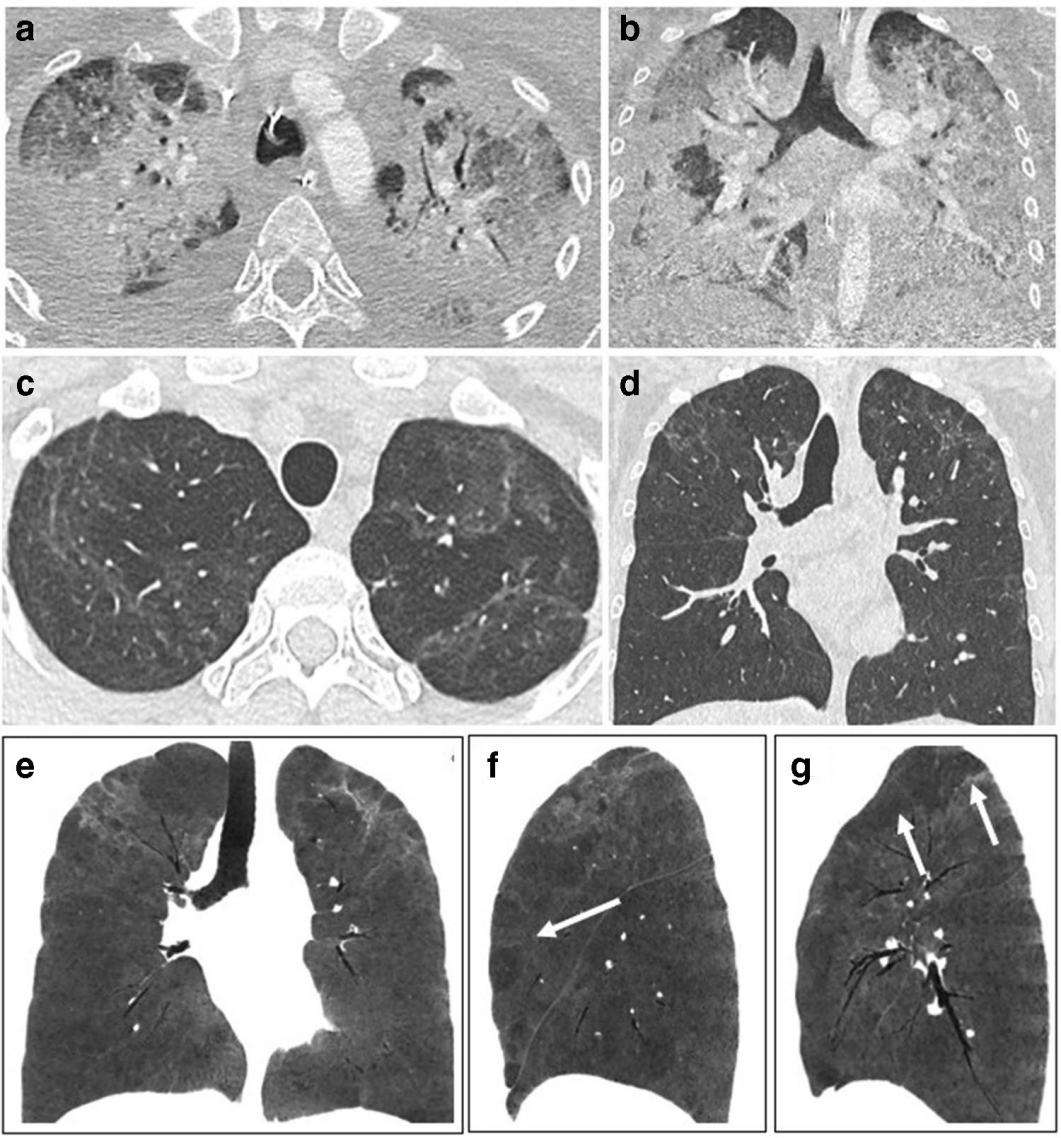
CT features at baseline, and 1-year follow-up, in a 30-year-old woman who developed severe COVID-19 pneumonia (intubation, 40 days in ICU). a, b Baseline CT showing extensive ground glass opacities (GGOs) and consolidation. c–g 1-year CT follow-up. Residual GGO, with a linear shape, is mainly seen in the upper lung, suggestive of organizing pneumonia at a late phase. There is no bronchial dilatation, signs of architectural distortion, or honeycombing, as confirmed by minimum intensity projections (minIP) (e, f, g). The sagittal minIP reformations (f, g) demonstrate plurilobular areas of decreased attenuation (arrows). This mosaic attenuation pattern might be due to either residual small airway disease or peripheral vascular obstruction. DLCO at 1 year was 64%
